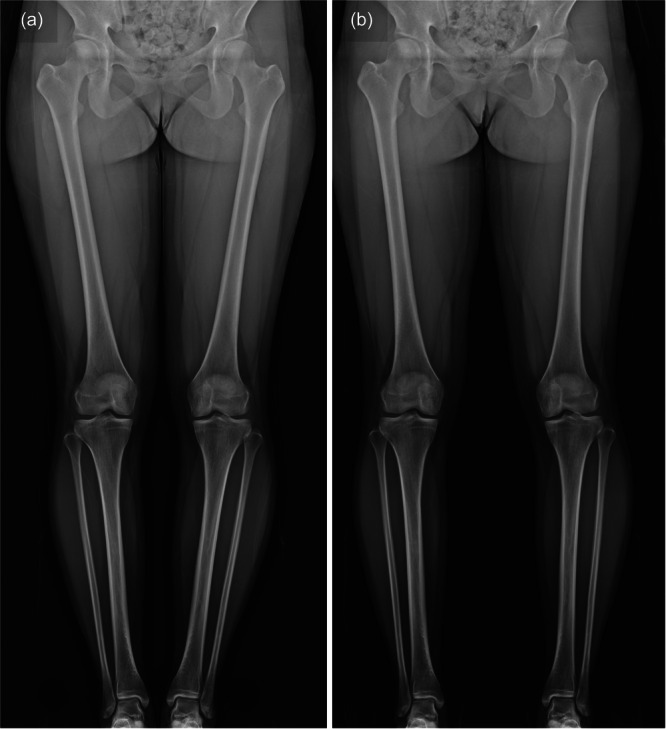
Full weight‐bearing coronal whole‐leg radiographs in the legs closed (a) and legs spread (b) standing with the patella centred on the femoral condyle.
